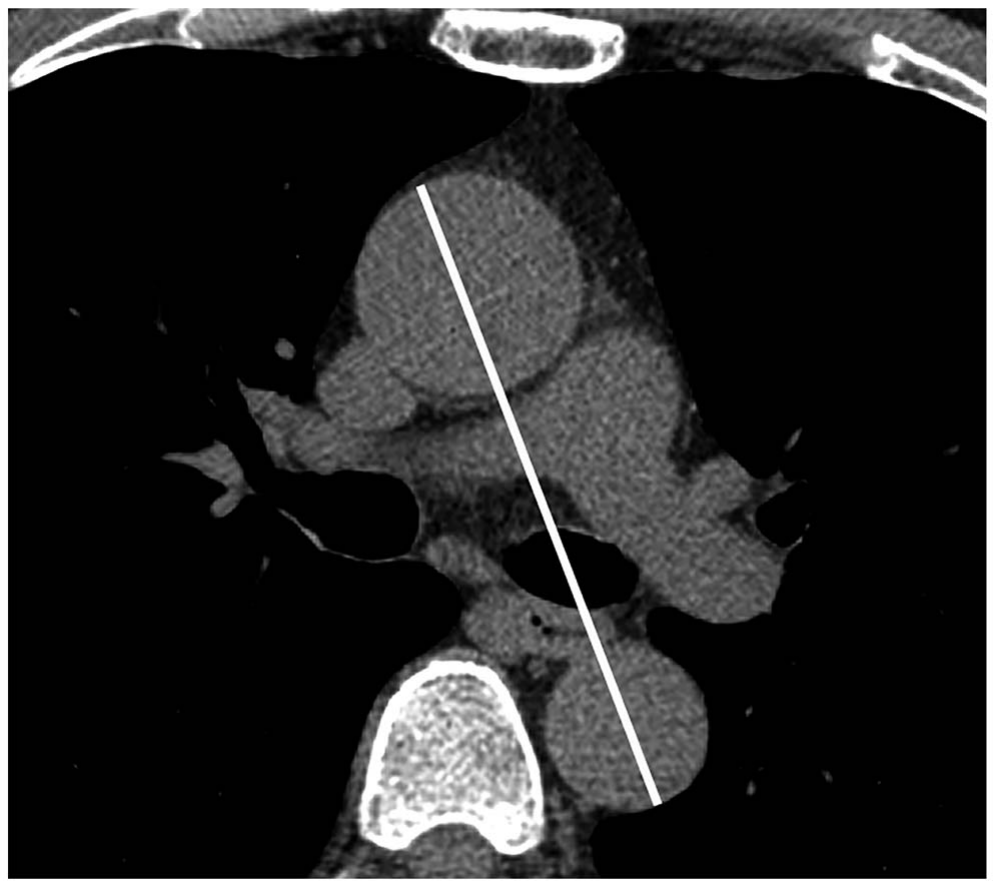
Measuring aortic unfolding. Aortic unfolding (white line) was defined as the longest distance between the ascending and descending aorta on a transaxial slice at the level of the pulmonary artery bifurcation on a selected coronary artery CT image.
